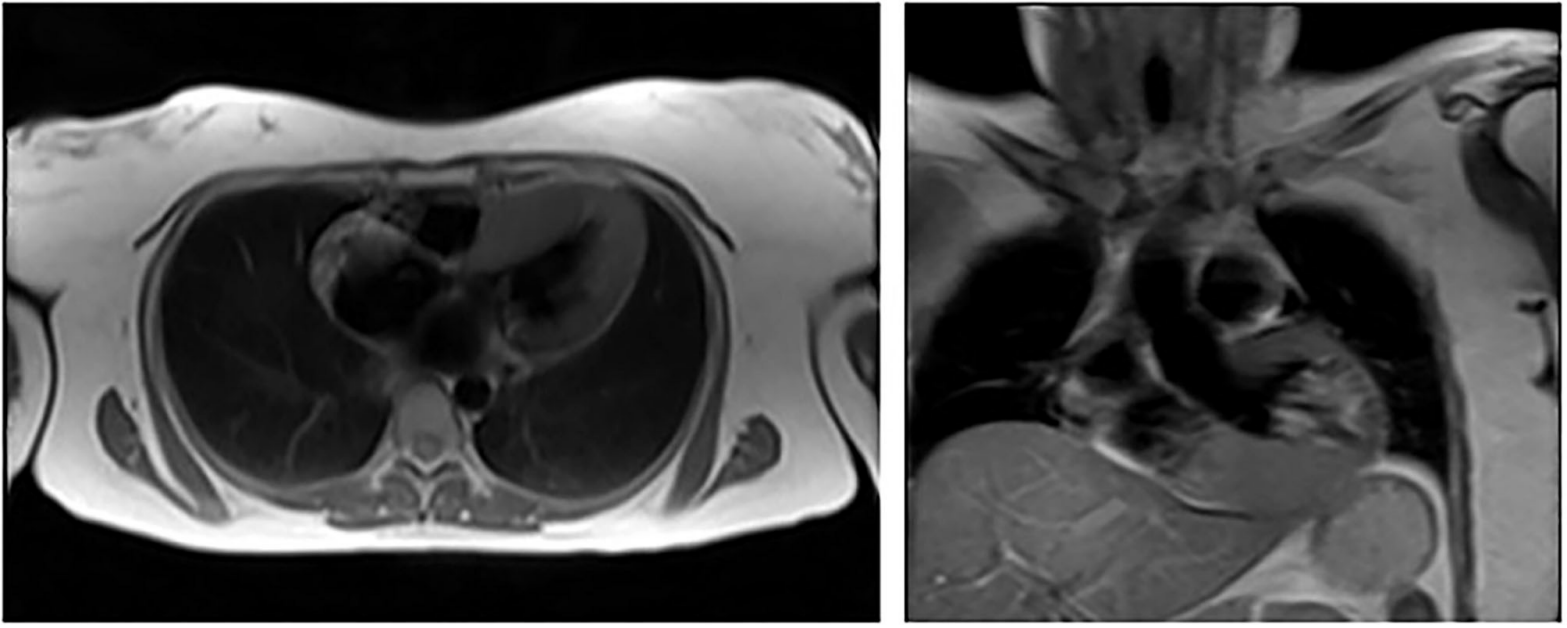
Cardiac MRI showing left ventricular hypertrophy in patient 2 (III – 16).
